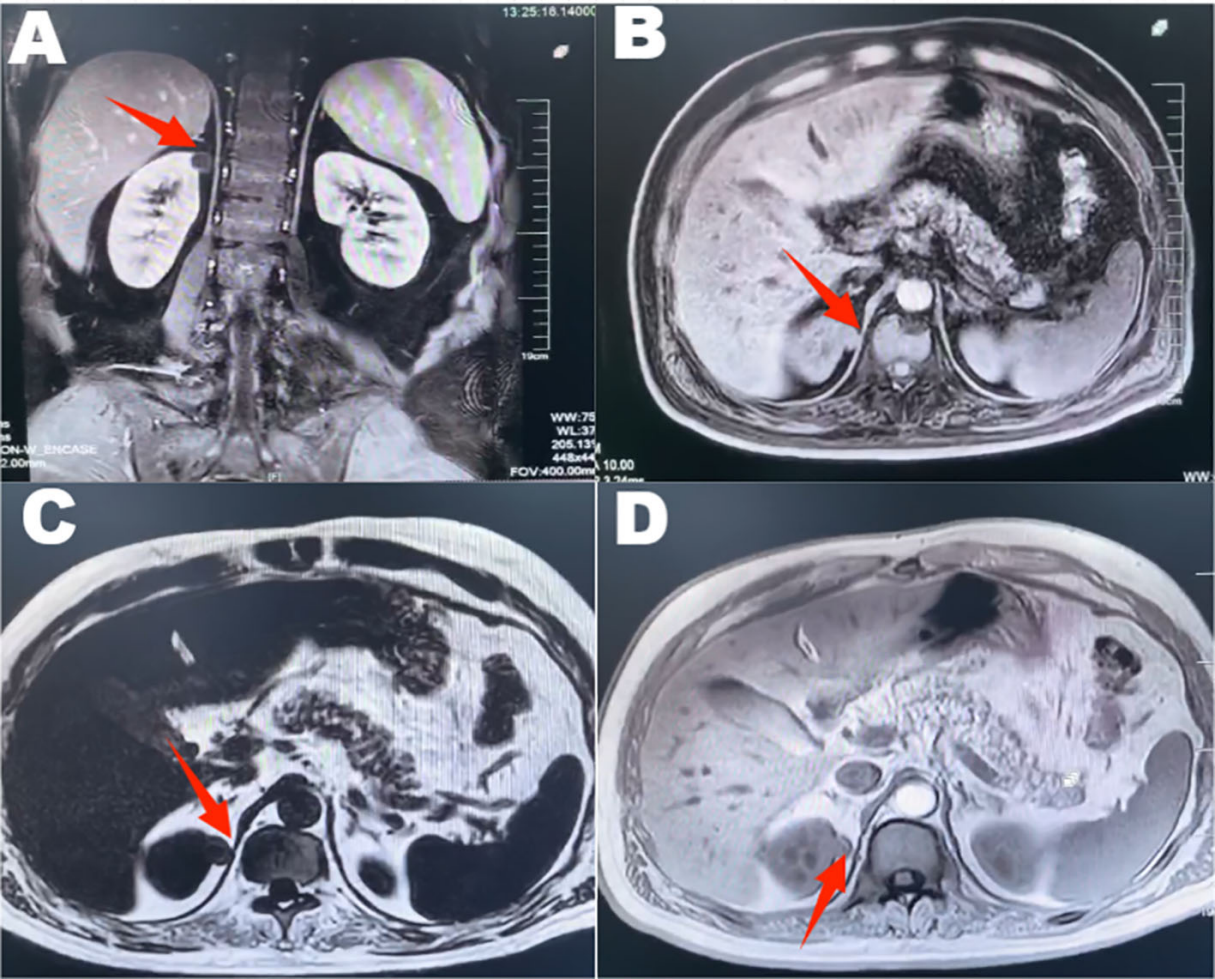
MRI reveals a protruding nodular lesion measuring approximately 1.6 cm at the margin of the right renal upper pole, exhibiting mildly prolonged T1 and T2 signal intensity. The lesion demonstrates low signal intensity on diffusion-weighted imaging and shows no significant enhancement on contrast-enhanced scans. This indicates the presence of an occupying lesion in the right upper renal pole, with associated hypoperfusion.
